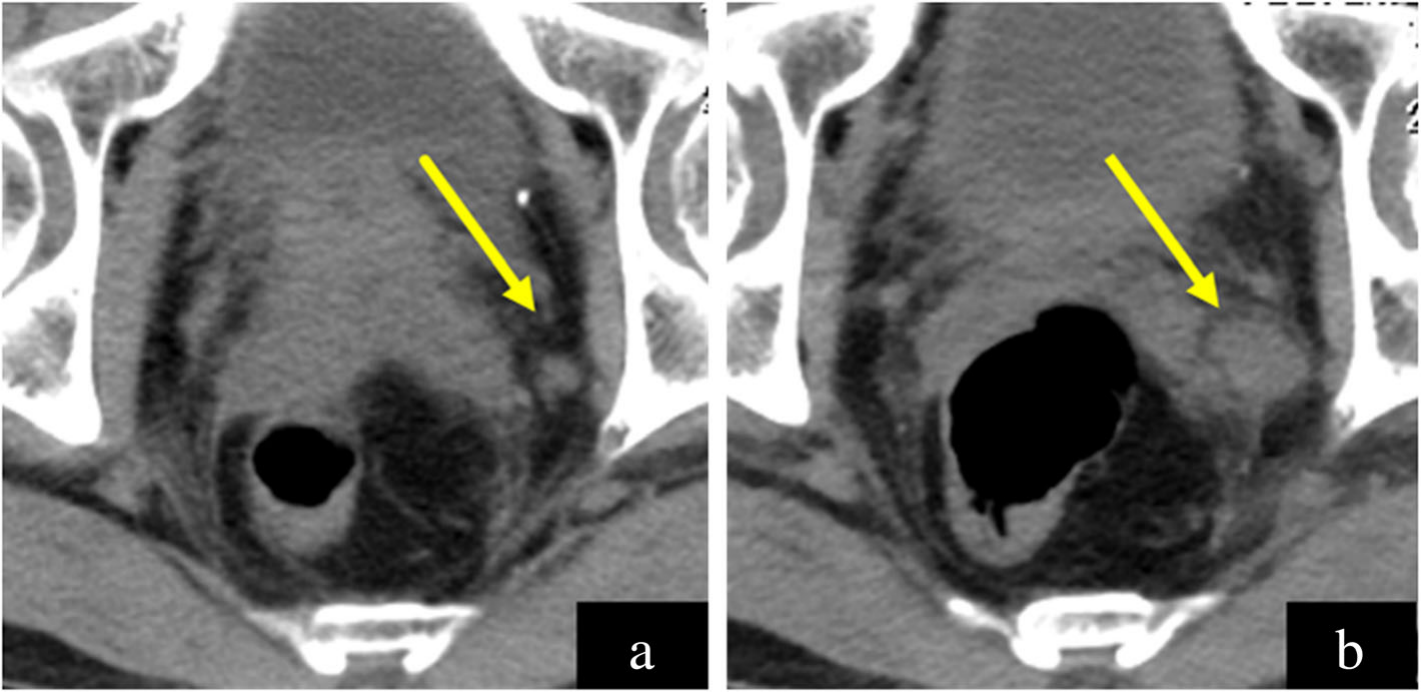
Follow-up computed tomography (CT) images after the primary surgery. a At 12 months after the primary surgery, CT shows a 4-mm mass at the left lateral region (arrow). b CT at 56 months after the primary surgery revealed enlargement of the indicated mass up to 20 mm (arrow)
